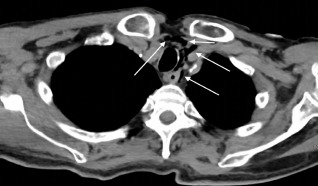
Computed tomography of the chest with contrast showing free air (arrows) adjacent to the trachea contained within the mediastinum secondary to the self-inflicted stab wound to the patient’s neck.
